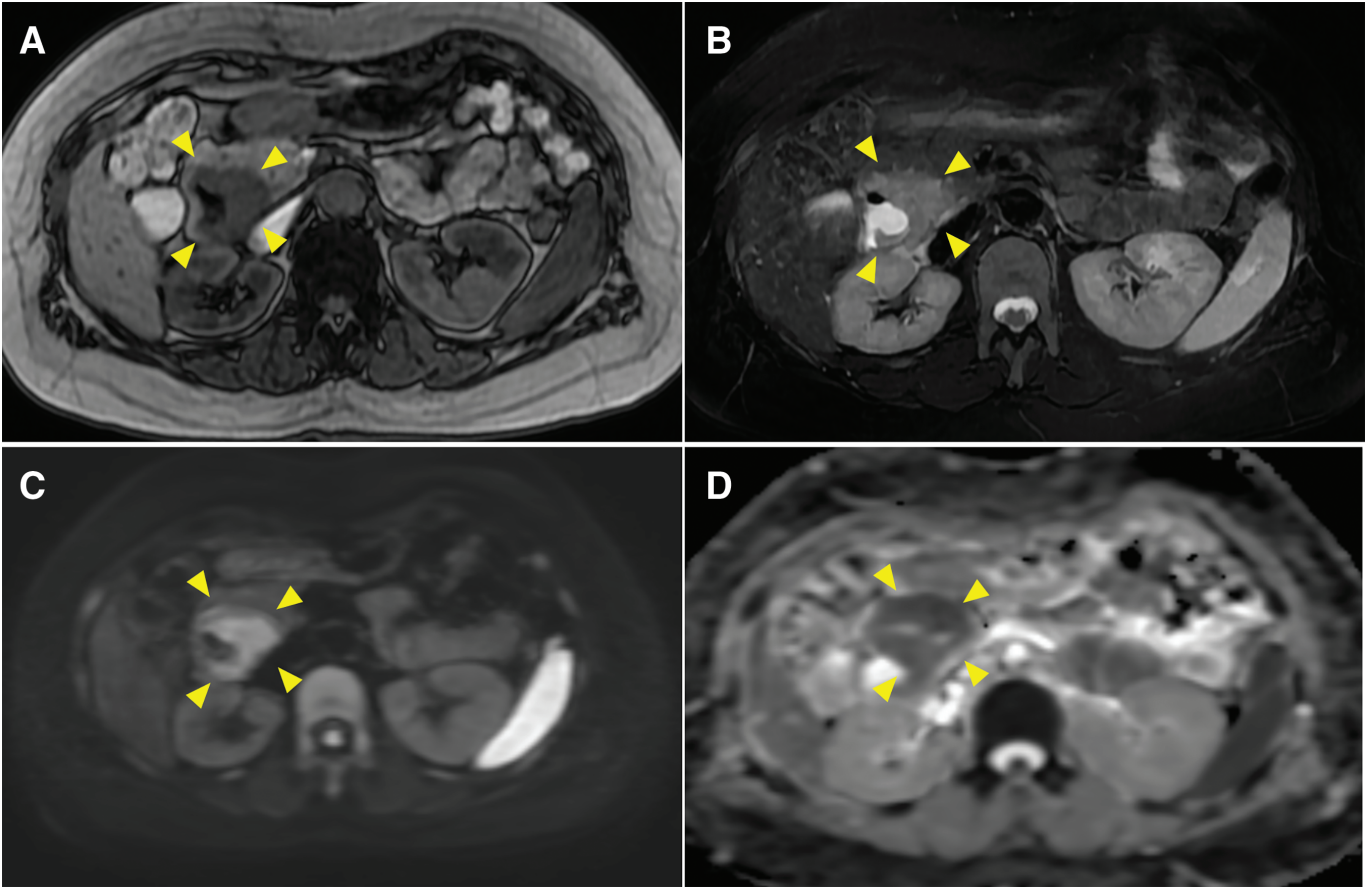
MRI findings. MRI shows a hypointense signal on T1-weighted images, a mildly hyperintense signal on T2-weighted images, high signal intensity on diffusion-weighted imaging, and low signal intensity on apparent diffusion coefficient map in the 2nd portion of the duodenum (yellow arrowheads). (A) Axial view, T1-weighted image; (B) axial view, T2 weighted image; (C) axial view, diffusion-weighted image; (D) axial view, apparent diffusion coefficient maps.
